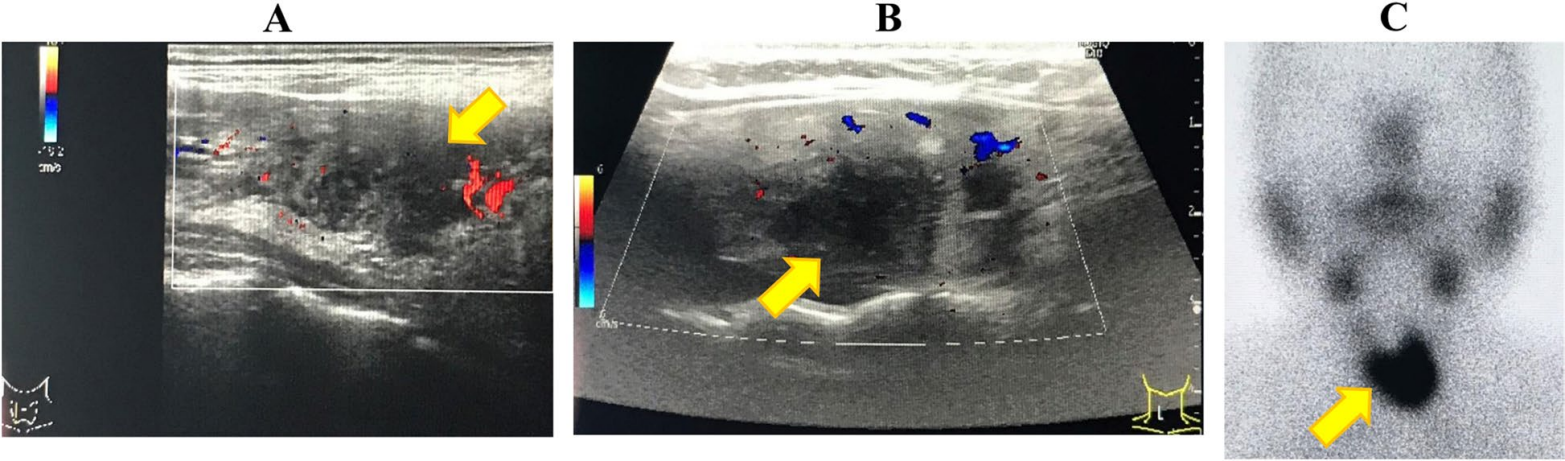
Thyroid ultrasonograms of two patients. Thyroid ultrasonograms of patient 1 (A) and patient 2 (B), indicating a focal hypoechoic area with decreased blood flow in the right lobe of the thyroid gland that coincided with the site of tenderness (arrow). Thyroid scintigram with ^99m^technetium pertechnetate of patient 2 (C) that was obtained at the recurrence of thyrotoxicosis shows the accumulation of an artificial radioactive element in the thyroid gland (arrow)
